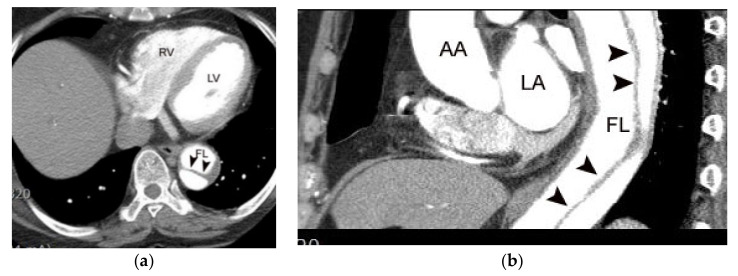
A case of type B aortic dissection identified on coronary CTA. Note the intimal flap within the descending thoracic aorta (arrowheads in (a,b)) RV, LA, LV, AA, FL indicate right ventricle, left atrium, left ventricle, ascending aorta, and false lumen, respectively.
